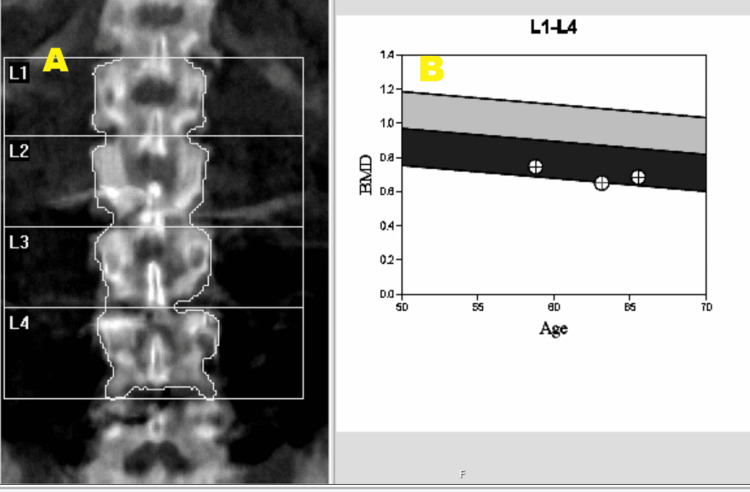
Evidence of osteoporosis affecting the lumbar vertebrae (L1-L4) Panel A: Latest DEXA scan study on patient assessing the lumbar vertebrae (L1-L4). BMD: 0.684 g/cm2; T-score:-3.3; Z-score:-1.5; Classified as: Osteoporosis Panel B: A graph depicts the serial trend of BMD results for the L1-L4 lumbar vertebrae. BMD: Bone mineral density; DEXA: Dual-energy X-ray absorptiometry
